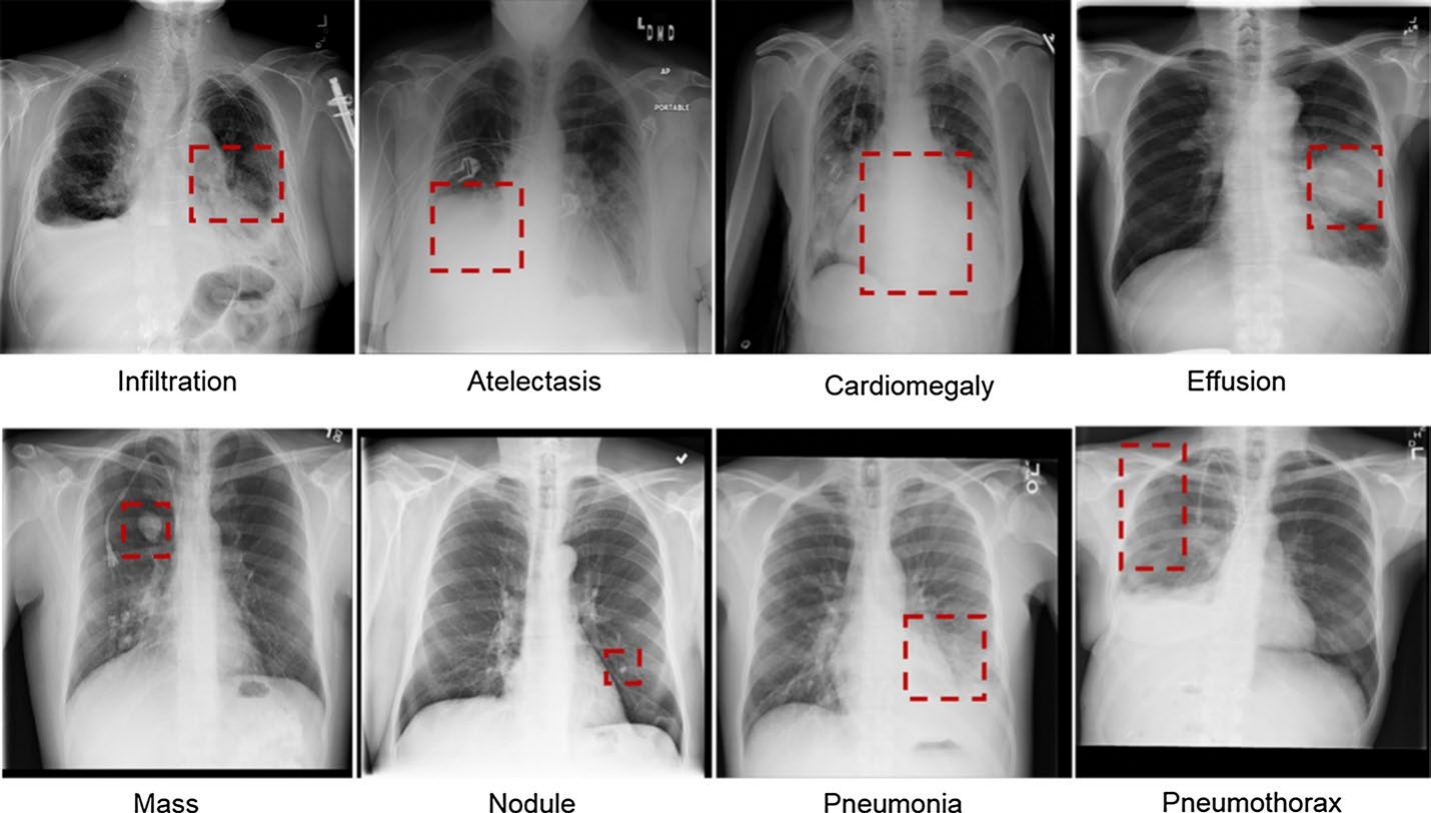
Eight common diseases such infiltration, atelectasis, cardiac hypertrophy, effusion, lumps, nodules, pneumonia, and pneumothorax observed in the chest radiographs
